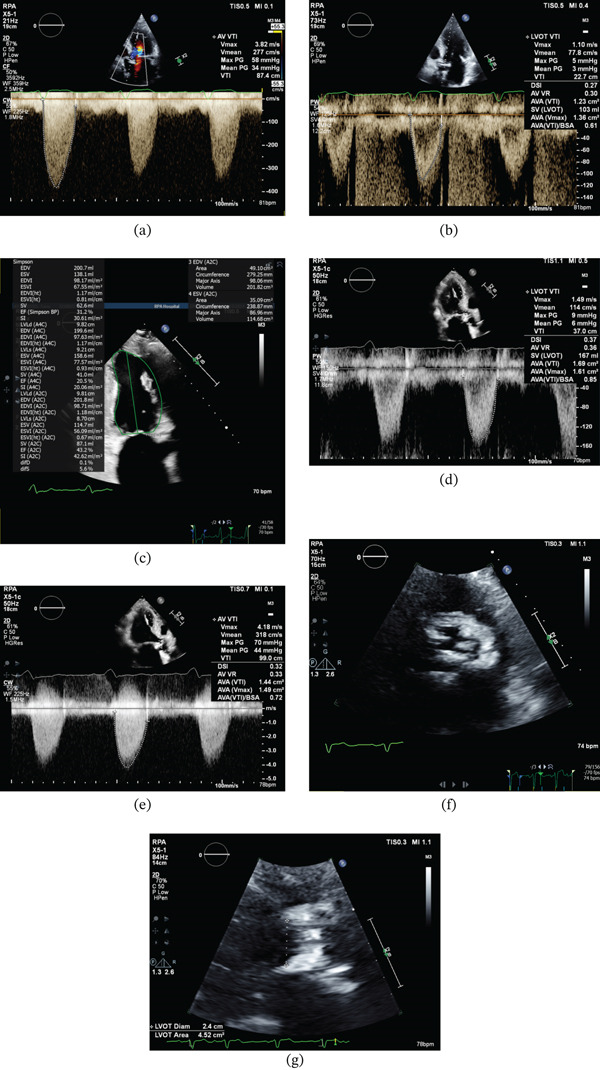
Available transthoracic and dobutamine stress echocardiographic images demonstrating discordant low‐gradient aortic stenosis. (a) Baseline/rest continuous‐wave Doppler across the aortic valve, showing Vmax 3.82 m/s, mean gradient 34 mmHg, and VTI 87.4 cm on a representative beat. (b) Baseline/rest LVOT pulsed‐wave Doppler, showing VTI 22.7 cm and Doppler‐derived stroke volume 103 mL on a representative beat. (c) Peak‐stress apical biplane Simpson assessment, showing SVi 30.61 mL/m^2^ and LVEF 31.2% on a representative frame. (d) Peak‐stress LVOT pulsed‐wave Doppler, showing VTI 37.0 cm and Doppler‐derived stroke volume 167 mL on a representative beat. (e) Peak‐stress continuous‐wave Doppler across the aortic valve, showing Vmax 4.18 m/s, mean gradient 44 mmHg, and continuity‐equation AVA 1.44–1.49 cm^2^ on a representative beat. (f) Two‐dimensional short‐axis transthoracic echocardiographic view demonstrating marked calcification of the aortic valve. (g) LVOT diameter measurement view demonstrating an LVOT diameter of 2.4 cm and LVOT area of 4.52 cm^2^. Representative still frames are shown. Displayed overlay values may differ slightly from the tabulated study‐summary values cited in the text because of beat‐to‐beat variability and tracing differences.
